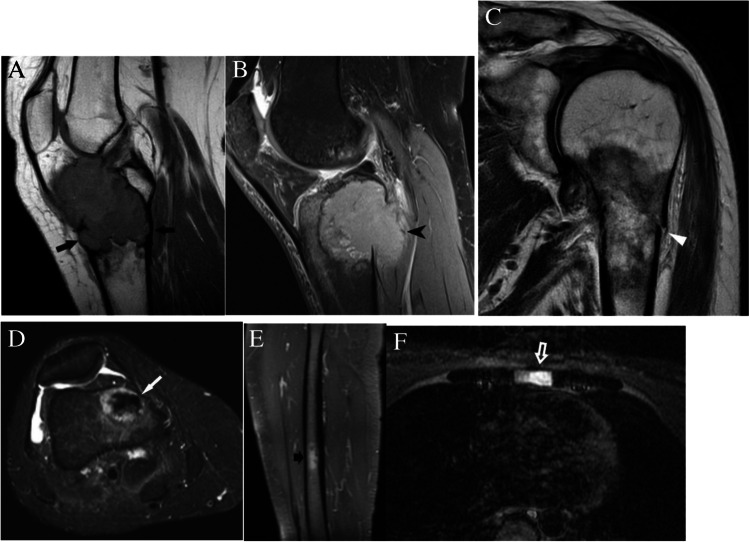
MRI assessment of concerning features. A Sagittal T1-weighted MR image shows a giant cell tumor in the proximal tibia with anterior and posterior cortical involvement (arrows). B Sagittal T2-weighted fat-suppressed MRI image shows a renal cell metastasis in the proximal tibia with soft tissue extension (pointed arrow) posteriorly. C Coronal T2-weighted MR image shows a lung cancer metastasis in the proximal humerus with pathologic fracture (arrowhead) of the lateral cortex. D Axial T2-weighted fat-suppressed MR image shows a low signal prostate cancer metastasis in the distal femur with peripheral halo (thin arrow) of high T2 signal. E Sagittal T1-weighted fat-suppressed post-contrast MRI image shows an enhancing breast cancer metastasis (fat arrow) in the mid shaft of the femur. F Axial T2-weighted fat-suppressed MR image shows a hyperintense metastasis (outlined arrow) in the sternum in a patient with breast cancer
